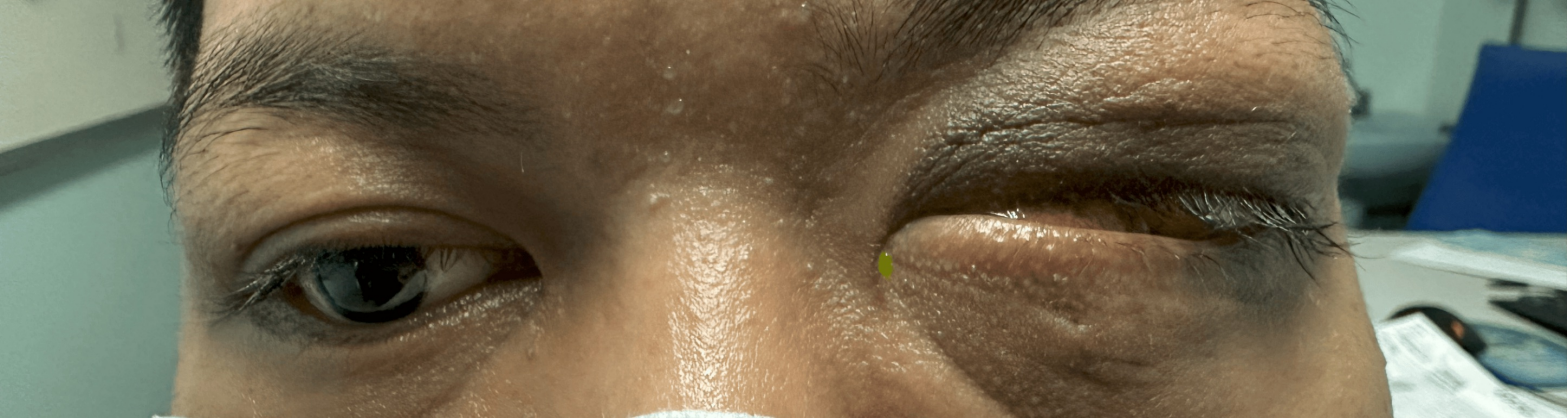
One month postoperative photograph showed reduced swelling, but the superior mass was noted to be slightly increased. The computed tomography (CT) scan revealed recurrence.
